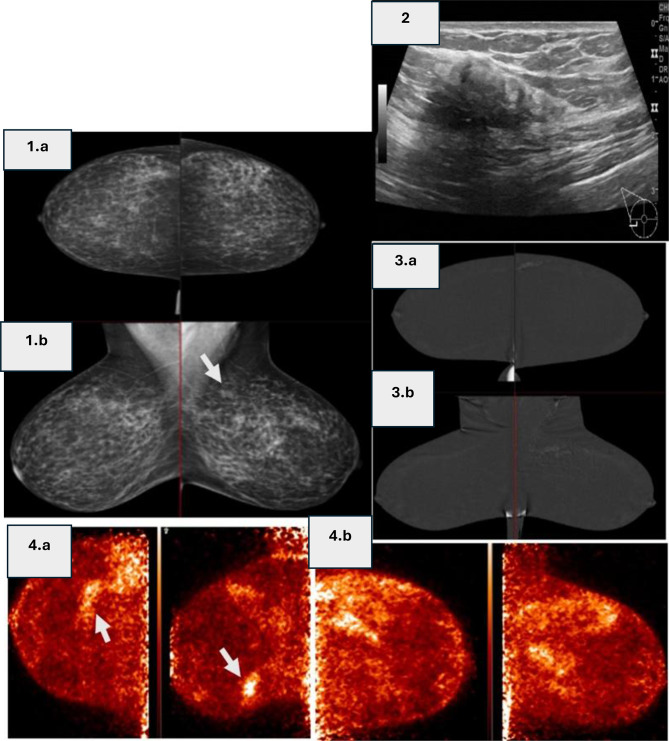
45 years female undergoing screening. (1. a) CC and (1. b) MLO Digital mammography (DM) show left upper quadrant single view asymmetry(arrow). (2) Grayscale ultrasound image of the right breast in the lower outer quadrant shows a heterogeneous, non-mass lesion with indistinct margins. (3.a) CC and (3. b) MLO Contrast-enhanced mammography (CEM) demonstrates linear non-mass enhancement in the upper outer quadrant of left breast (arrows), corresponding to asymmetry in DM, which was biopsy-proven for invasive lobular carcinoma, Yet no abnormal enhancing lesions at right side. (4.a) Right CC and MLO and (4. b) Left CC and MLO Positron emission mammography (PEM) of the same breasts shows corresponding left breast FDG-avidity, as well as FDG-avidity at the right side lower outer quadrant (arrow) corresponding to the abnormal area seen in U/S which warranted further biopsy. Yet, biopsy from the right lesion demonstrated PASH
